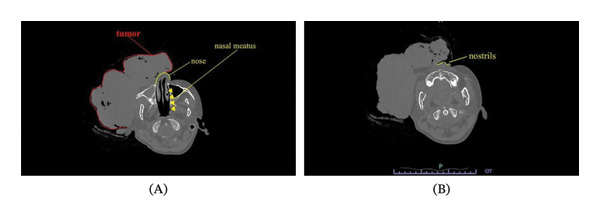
Preoperative CT scans of the patient. (A) The tumor had infiltrated the right orbit and maxillary sinus. The nasal meatus was open, and the upper airway was intact. (B) As the image shows, even though the patient’s nostrils were open, the tumor had grown right in front of the nose, meaning nasal intubation was not feasible.
